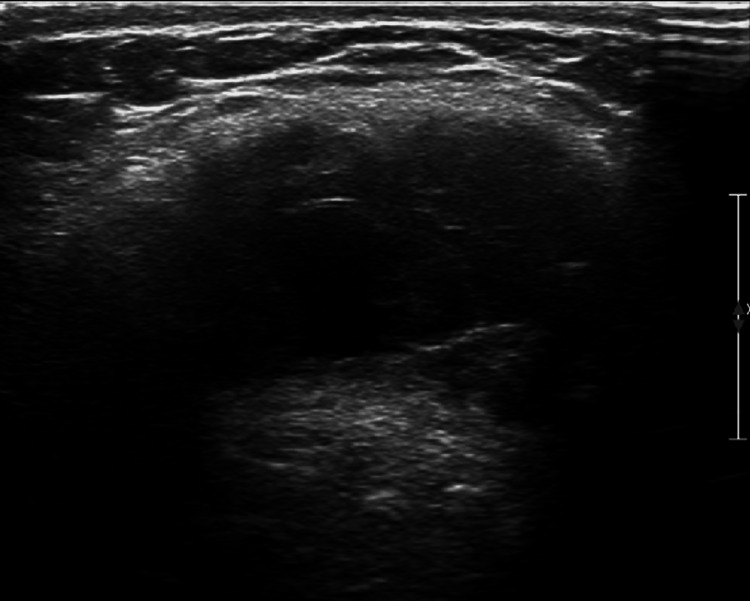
Ultrasonography of neck mass (transverse view).
